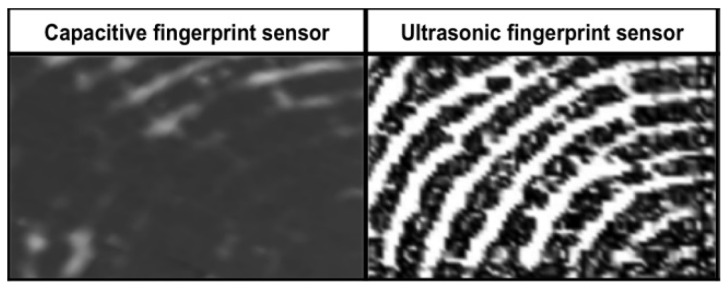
Fingerprint capture from capacitive vs. ultrasonic sensors. Reprinted with permission from Ref. [33] 2016, IEEE.
